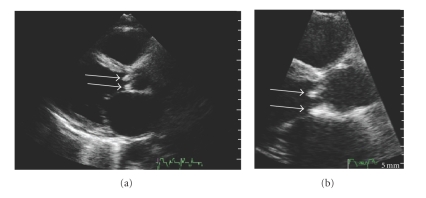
Transthoracic echocardiographic examination: parasternal long axis view. The arrows indicate two vegetations on the ventricular side of the aortic valve cusps (right and noncoronary).
